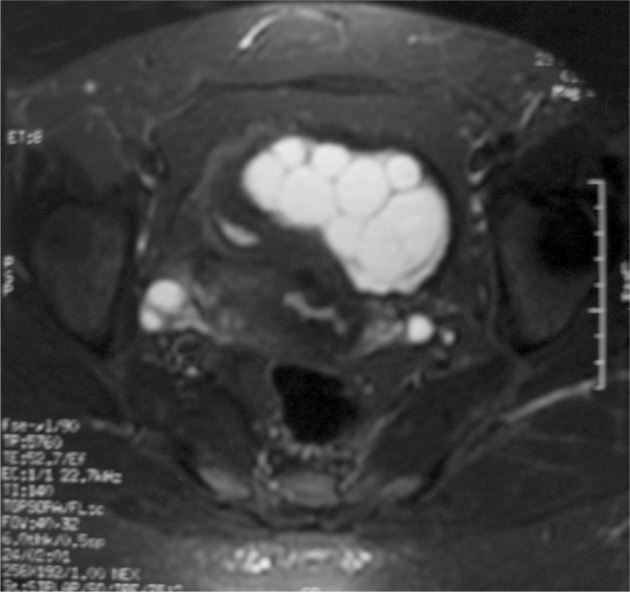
Pelvic MRI revealed a septated, lobulated and contrast enhancing cystic mass that is hyperintense on T2 and hypointense on T1 FLAIR sequences.
